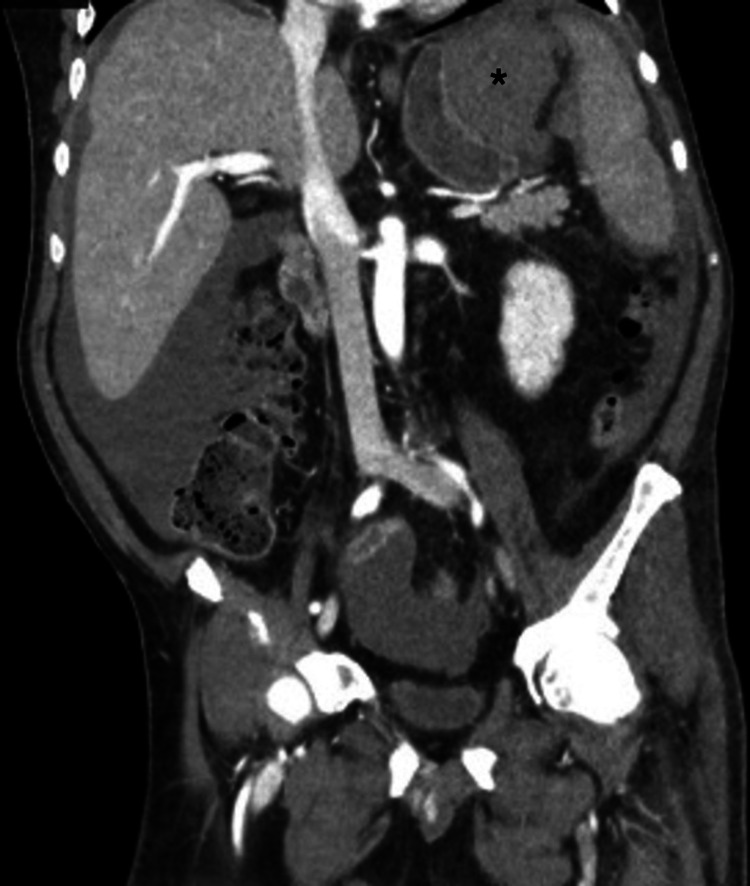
CT angiography image with gastric intramural hematoma marked with (*)
